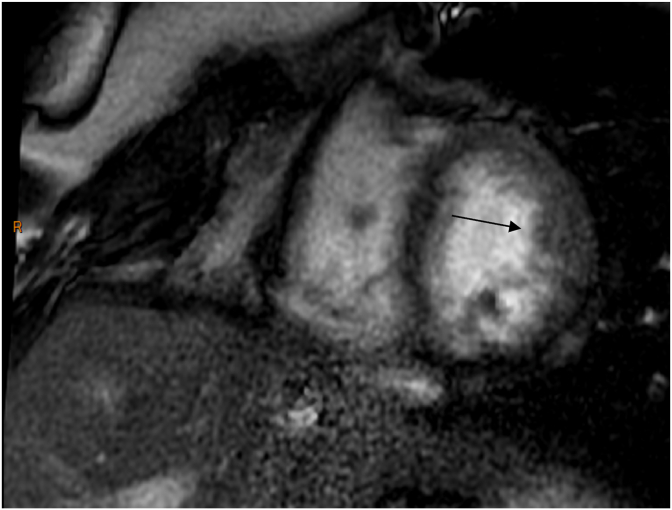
FIESTA MRI sequence: Cardiac short-axis slices showing left ventricle myocardial thickness
